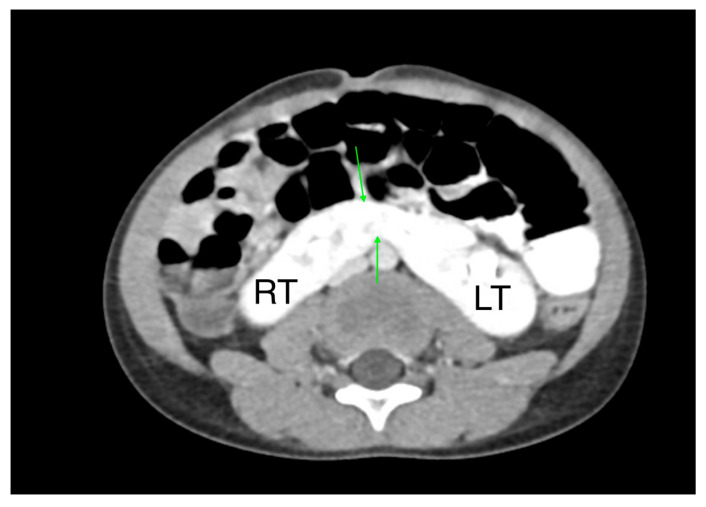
A CT scan reveals a horseshoe kidney, with fusion of the lower poles indicated by green arrows.
